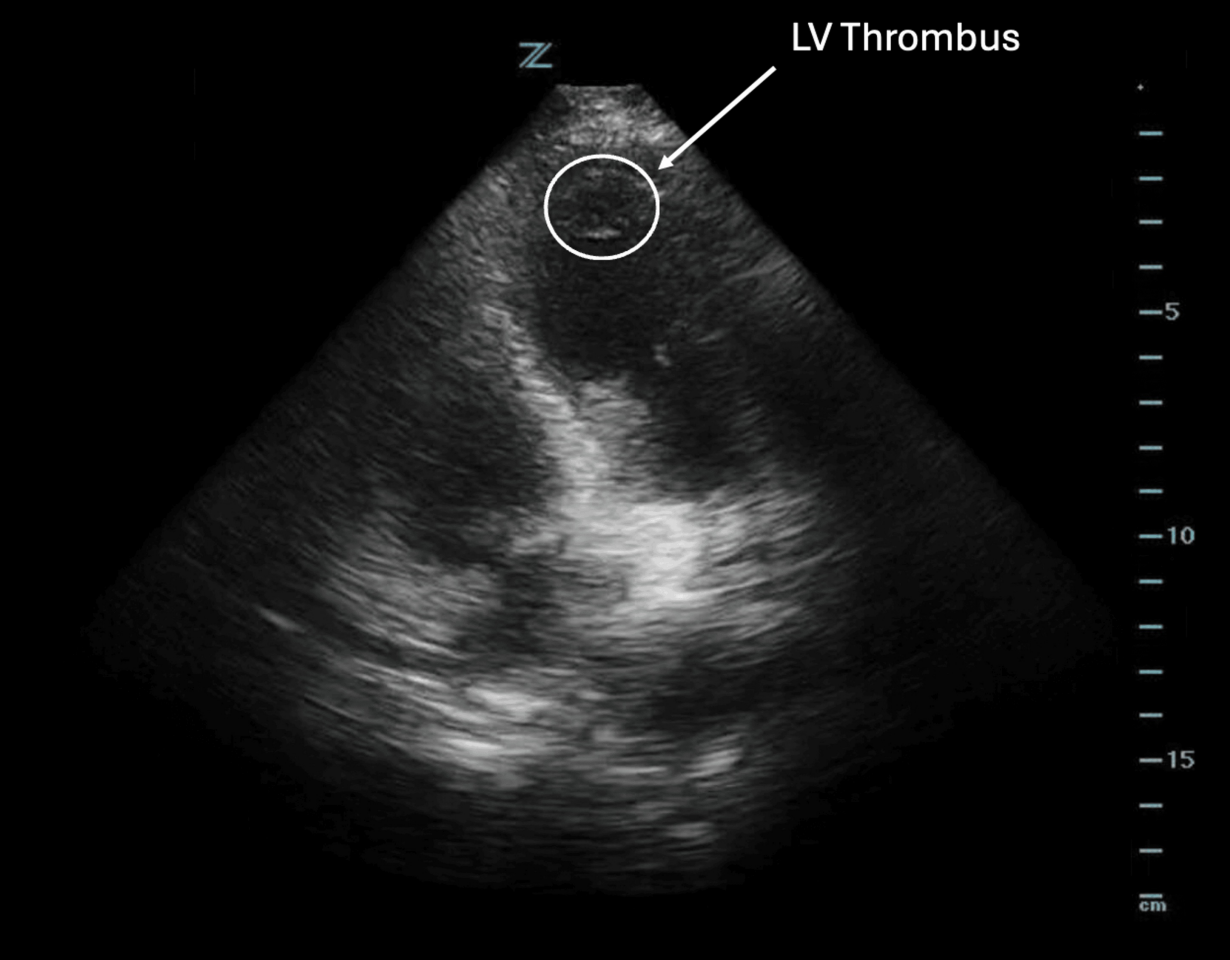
An apical four-chamber view of the left ventricle in Case 1, obtained using a phased array probe, demonstrating a left ventricular thrombus (outlined in white circle).
